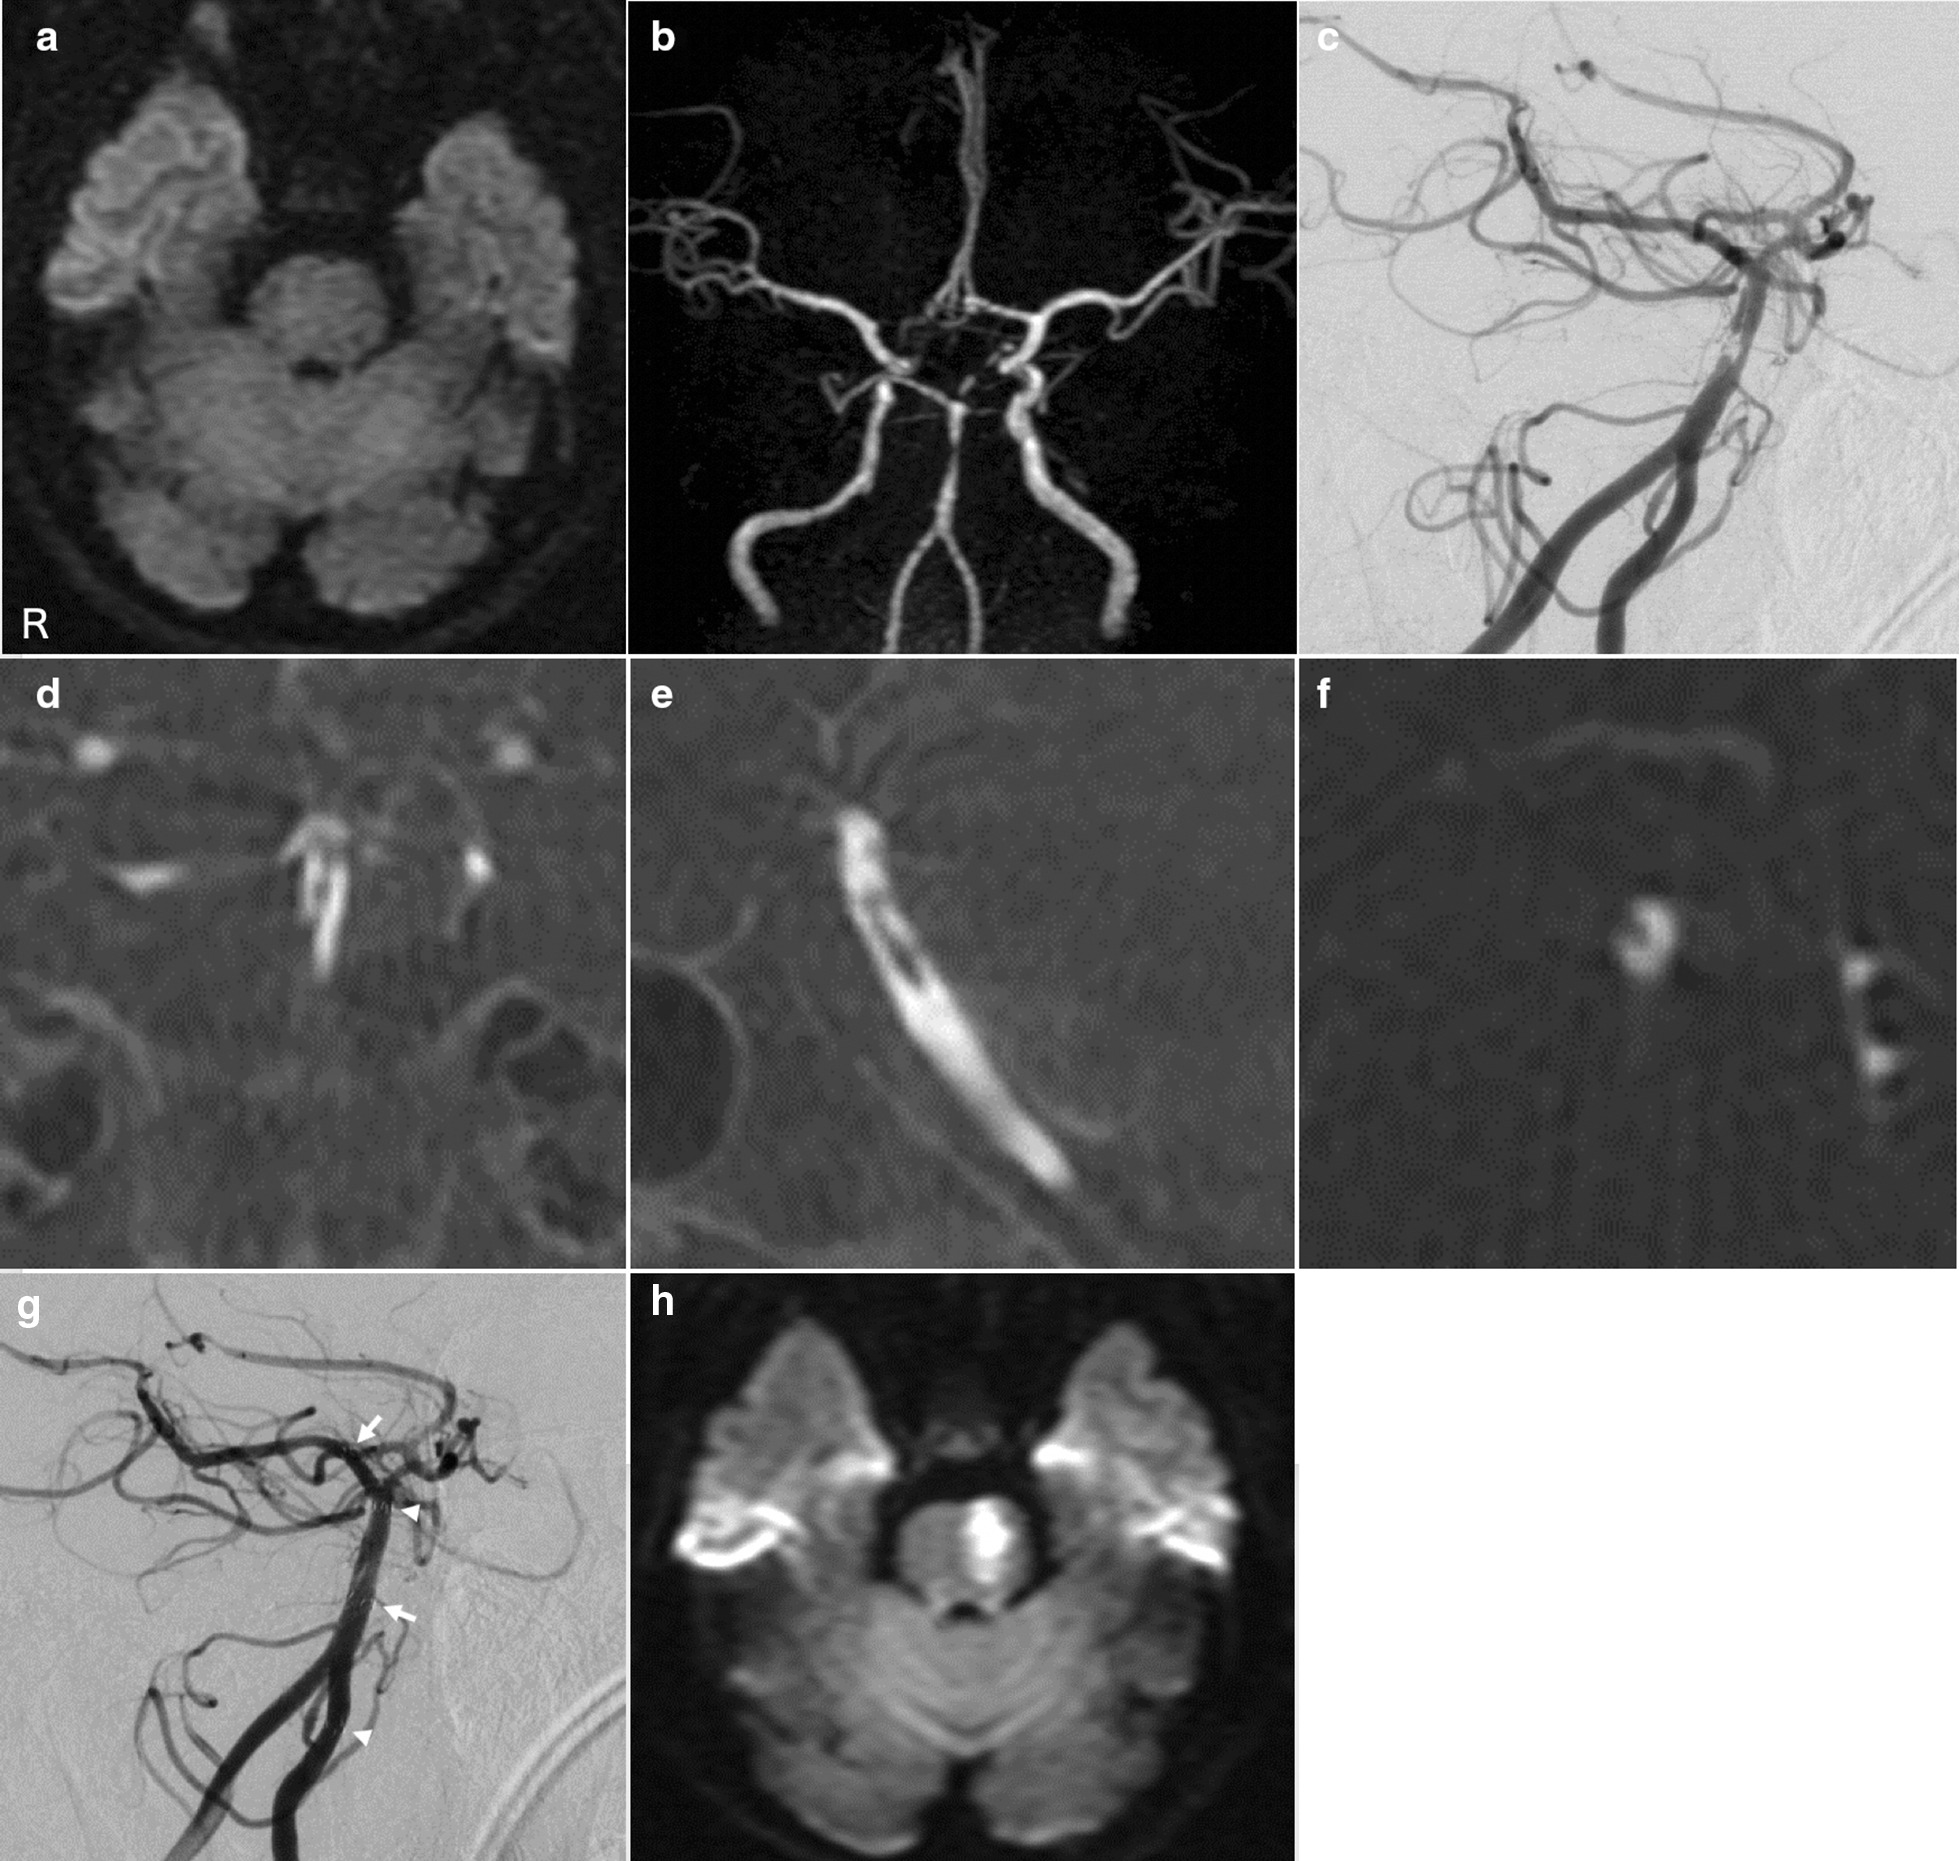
a Brain magnetic resonance imaging (MRI) at 90 minutes from symptom onset showing no high-intensity area on diffusion-weighted imaging (DWI). b Brain magnetic resonance angiography showing stenosis in the middle portion of the basilar artery (BA). c Cerebral angiography (45° right-anterior oblique view) showing intimal flap and double lumen in the middle and distal portions of the BA. Maximum intensity projection images of 3D rotational angiography showing double lumen of the BA in the coronal view (d), sagittal view (e), and axial view (f). g Cerebral angiography (45° right-anterior oblique view) after stenting. Enterprise VRD 4.5 × 22 mm^2^ (arrow: distal and proximal marker) and 4.5 × 28 mm^2^ (arrowhead: distal and proximal marker) was deployed from the P1 portion of the right posterior cerebral artery to the V4 portion of the left vertebral artery. h Brain MRI on day 6 showing left-sided pontine infarction on DWI
